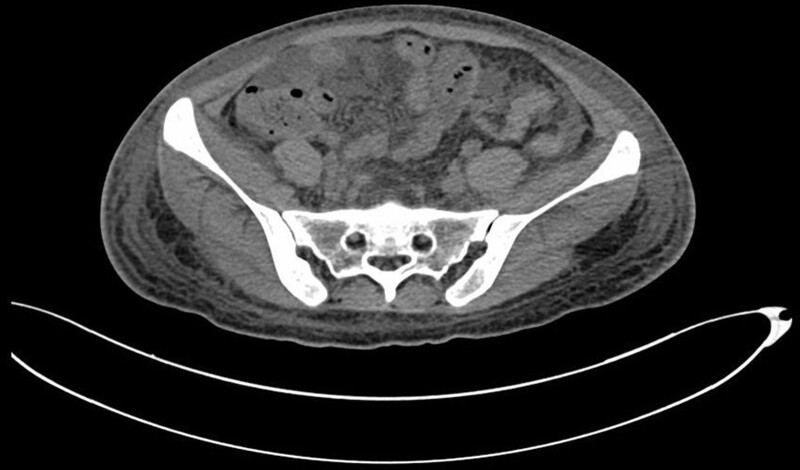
Abdominal computed tomography on the 9th day of admission showing no abnormalities in the uterus and bilateral adnexal areas.
